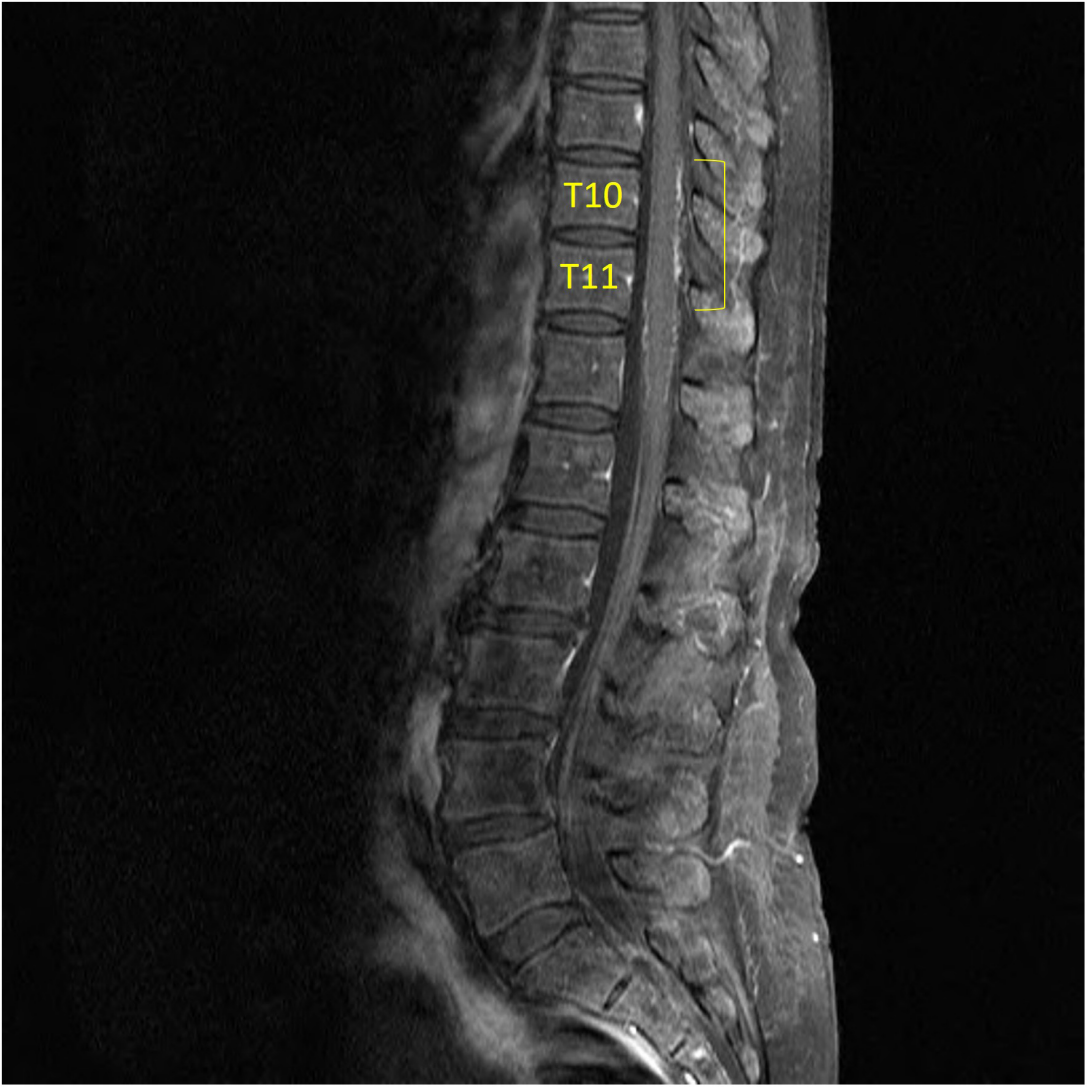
MRI whole spine outlining subtle posterior meningeal enhancement at T10 and T11.
